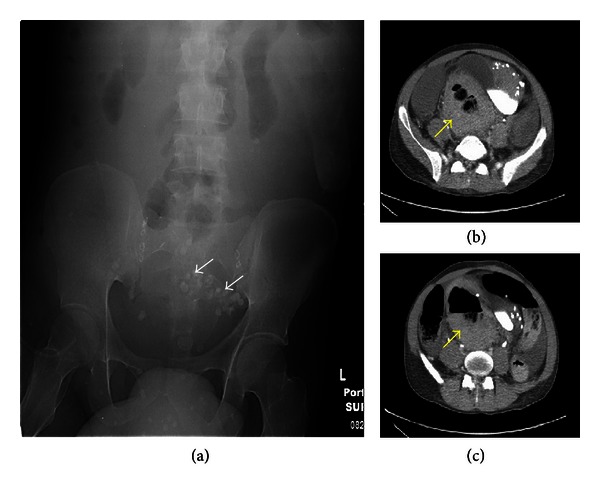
(a) Kidneys, Ureters, and Bladder (KUB) imaging shows numerous venous calcifications consistent with phleboliths from vascular abnormalities in pelvic area (white arrows). (b) and (c) Computed Tomography (CT) scan of the abdomen and pelvis shows markedly thickened colonic (rectal) wall (yellow arrows).
